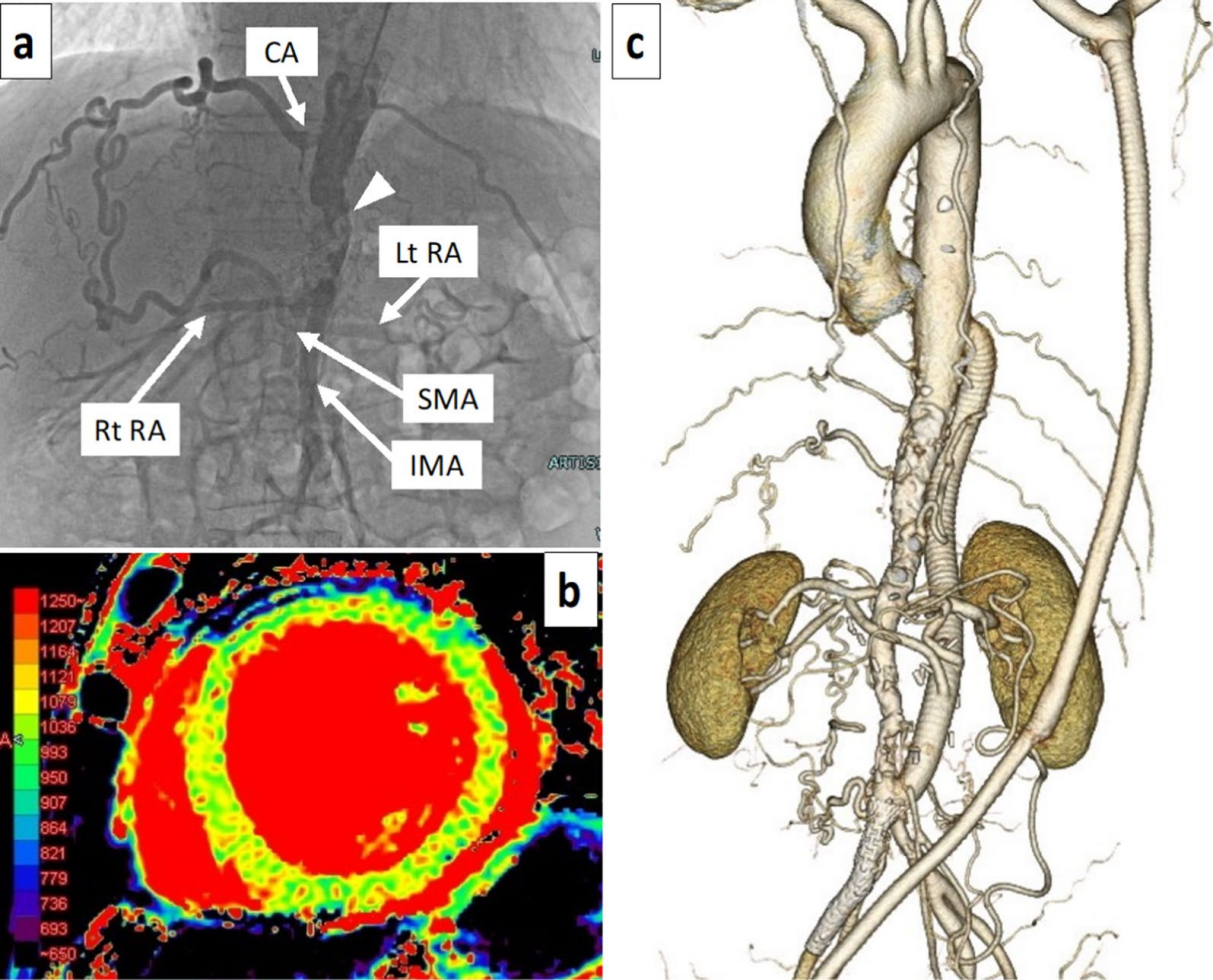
Preoperative aortography, magnetic resonance imaging, and postoperative computed tomography. a Aortography showing the superior mesenteric artery (SMA) imaged by the well-developed collateral circulation from the celiac artery (CA). Bilateral renal arteriography was delayed. Severe stenosis is observed in the epigastric aorta of the renal artery (arrowhead). b T1 mapping images on visceral MRI shows a slight increase in the T1 value of the left ventricular wall and no fibrosis progression on the left ventricular wall. c Postoperative 3D-CT showing that the descending thoracic aorta-abdominal aorta bypass and bilateral renal artery bypass were patent
